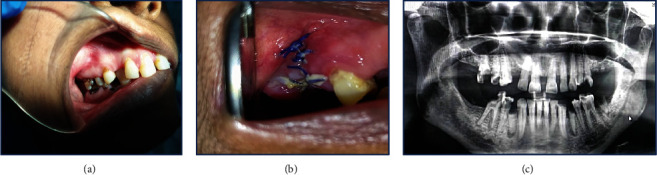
(a) Clinical presentation of the case, diffuse swelling adjacent to the grossly carious teeth on the vestibule, (b) postsurgical healing after 8 days, and (c) the orthopantomography of the patient showing minimal radiolucency on the root apices masked by the maxillary sinus.
